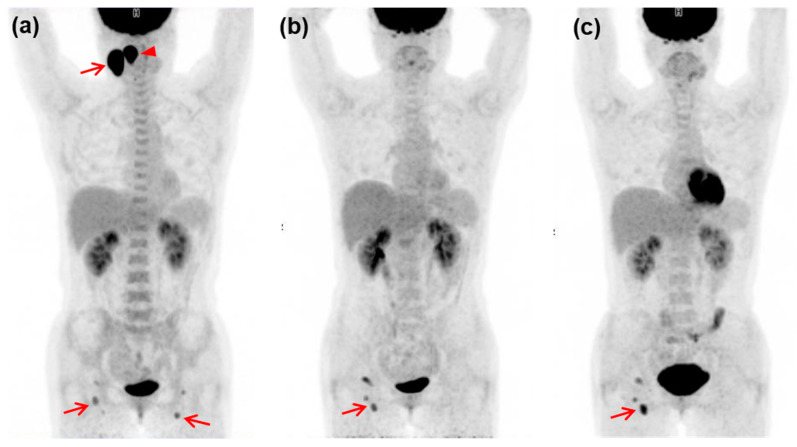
A 43-year-old female patient with PTCL-NOS, with IPI score of 1. B-PET image (a) shows intensely increased FDG uptake in the right palatine tonsil (SUVmax 12.8, arrowhead) and right cervical level II lymph node (SUVmax 17.6, arrow). In addition, lesser intense FDG uptake was noted in right cervical level I–II and bilateral inguinal nodes (arrows). Both i-PET (b) and e-PET (c) images were positive (Deauville score of 4), with persistently increased FDG uptake in the right inguinal nodes (arrows). The patient had a PFS of two months and an OS of nine months.
